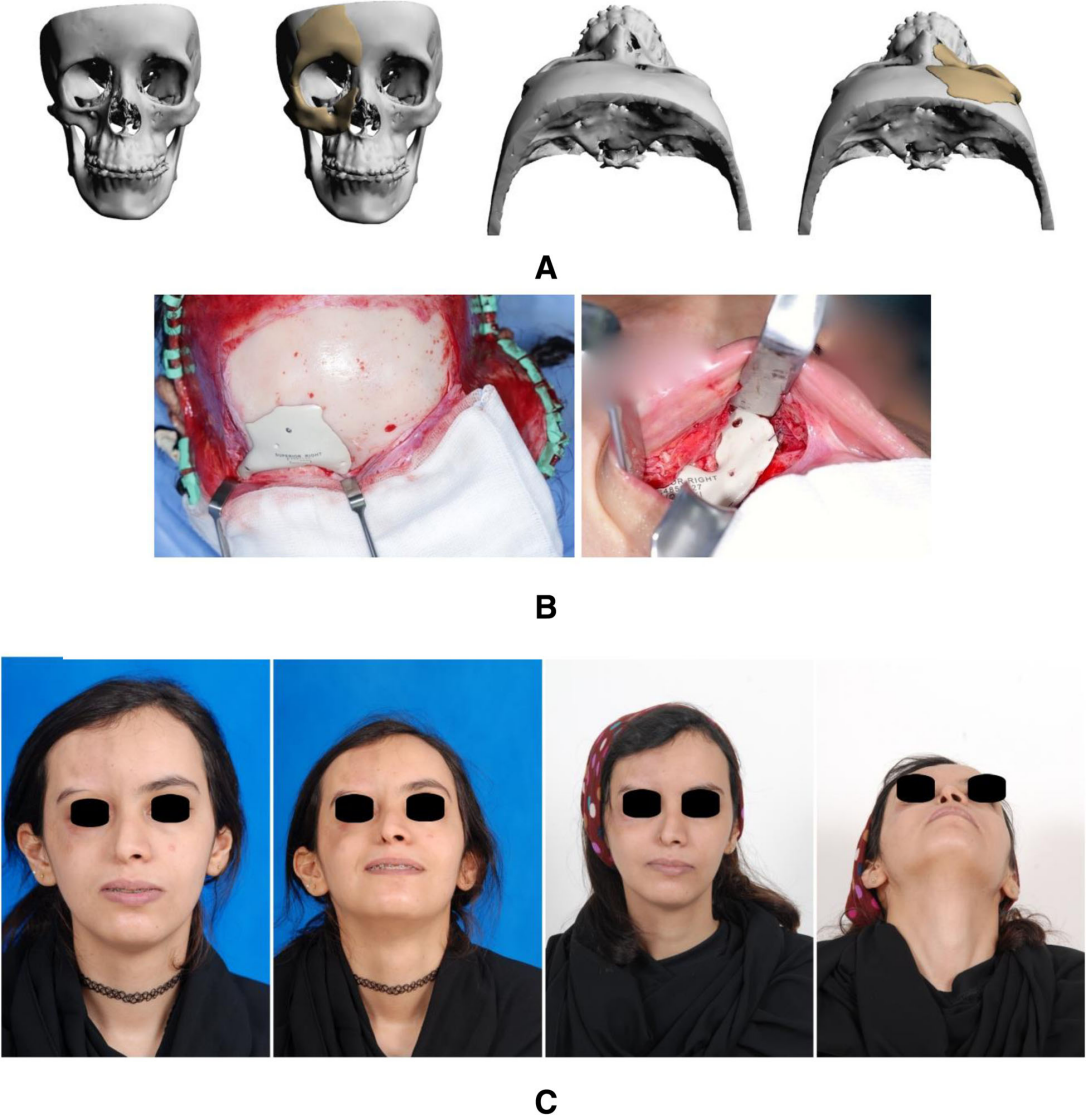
Parry-Romberg syndrome case. a 3D reconstruction of the CT showing the defects and the planned implants. b Intraoperative views of the implants. c Pre- and postoperative photographs of the patient
